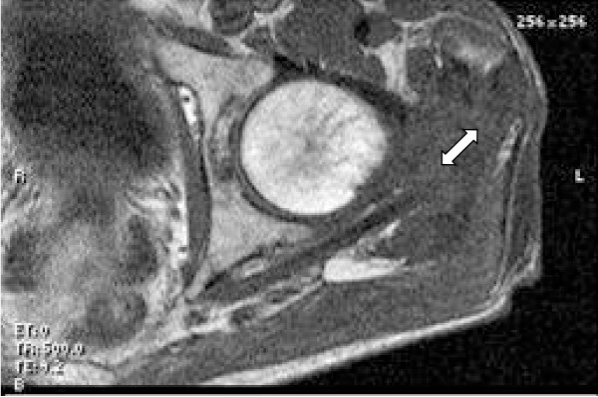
MRI of the left thigh showing loculated fluid collection (↔) contiguous with the gluteus medius and pyriformis muscles.
